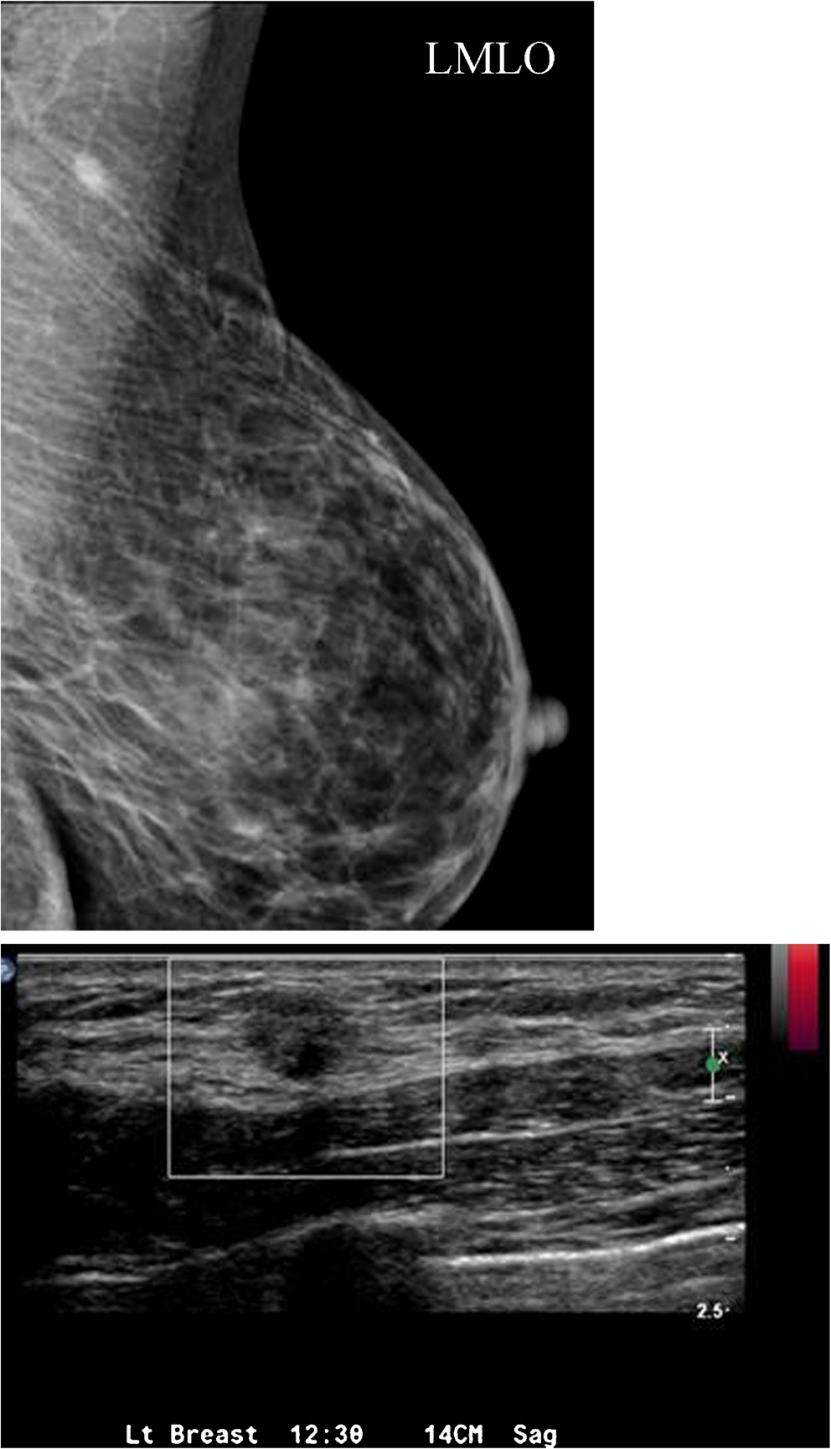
A 50-year-old female with focal 1-cm asymmetry in the left axilla region. a MLO views demonstrate left axillary irregular asymmetry, which persisted on spot compression. b Ultrasound of the left axilla demonstrated a 12-mm irregular hypoechoic hypovascular mass in the left axilla. Ultrasound-guided core needle biopsy was performed. Final diagnosis: granular cell tumour
